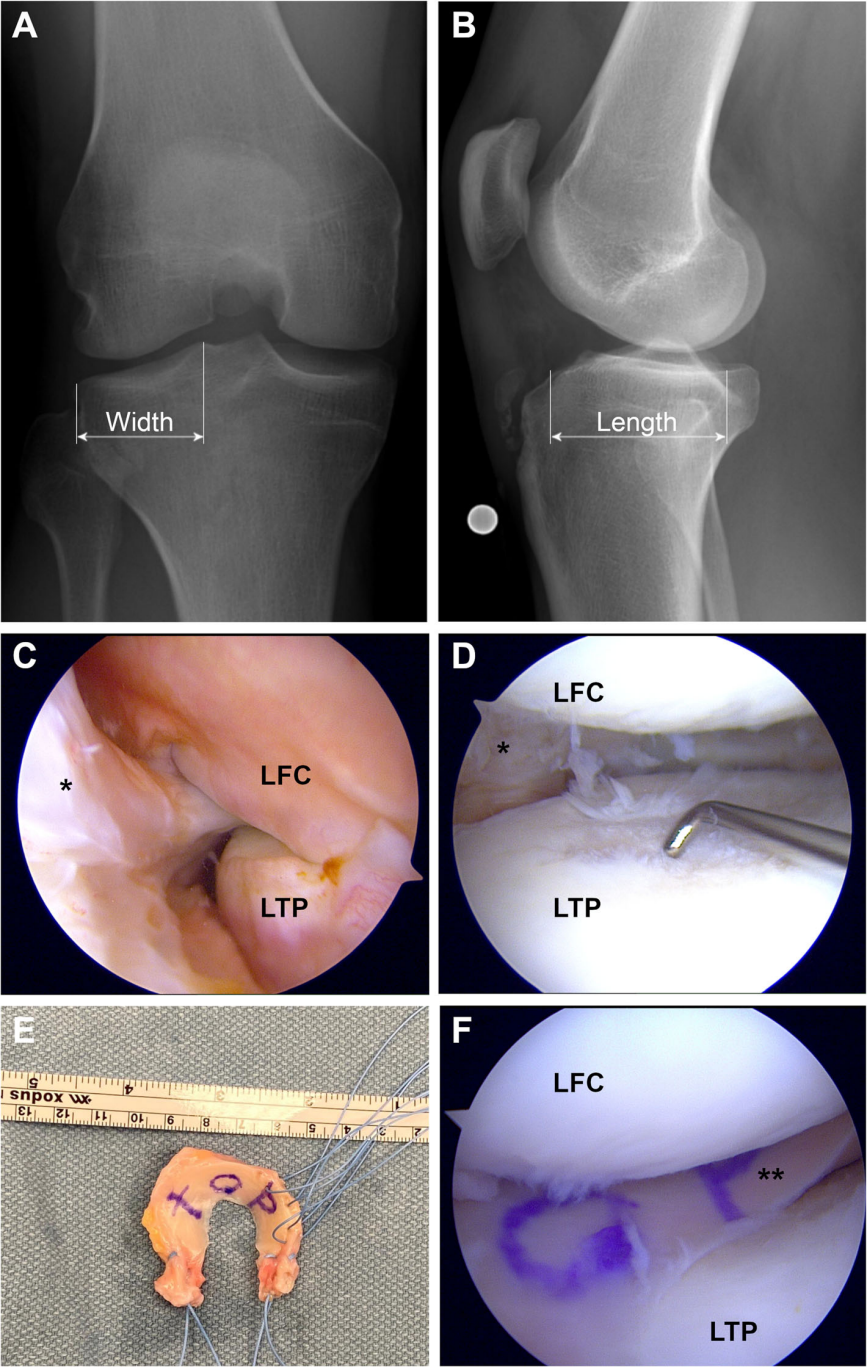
Lateral meniscal allograft transplantation (MAT) by suture-only fixation technique in a patient having undergone subtotal lateral meniscectomy without rim preservation (right knee). Preoperative a) anterior-posterior (AP) and b) lateral radiographs for sizing of the meniscal allograft. The width is measured on the AP radiograph as the distance from the lateral border of the tibial plateau to the apex of the lateral tubercle of the intercondylar eminence. The length is determined as 70% of the measured length of the lateral tibial plateau [63]. c) Arthroscopic view of the lateral gutter. Note the absence of meniscal tissue between the lateral femoral condyle (LFC) and the lateral tibial plateau (LTP); d) Arthroscopic view of the lateral compartment with grade 2 femoral and tibial cartilage lesions; e) Lateral meniscal allograft after preparation and application of the fixation sutures; f) Arthroscopic view of the lateral compartment after lateral MAT; *,Remnant of the native lateral meniscus; , Lateral meniscal allograft in situ
